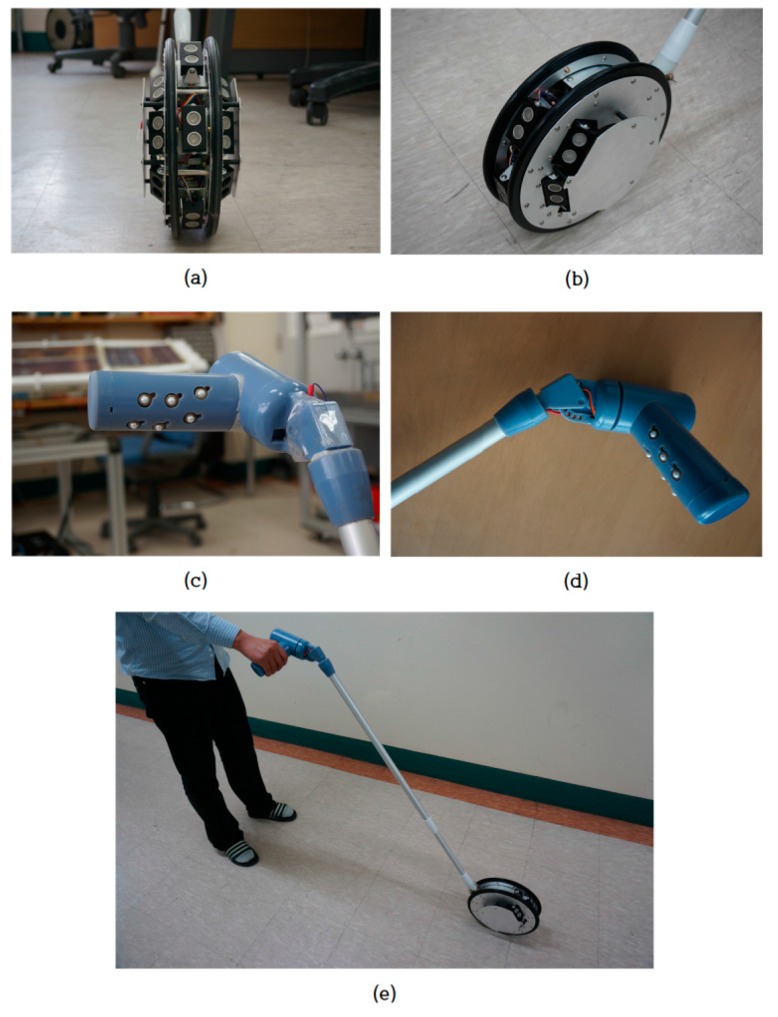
Assembled light-weight ETA with improved tactile display. (a) Front view; seven ultrasonic sensors are attached (2 × 3 for front and one for downward detection); (b) Side view; bearing-supported outer rim is rollable and the mechanism protects the sensors from an unexpected collision; (c) Implemented tactile display with 2 × 3 vibrotactile actuator to stimulate the corresponding fingers; (d) Grip-type tactile display enhances close contact of the user’s hand, and is adjustable to the posture of user’s hand; (e) User and the assembled ETA.
